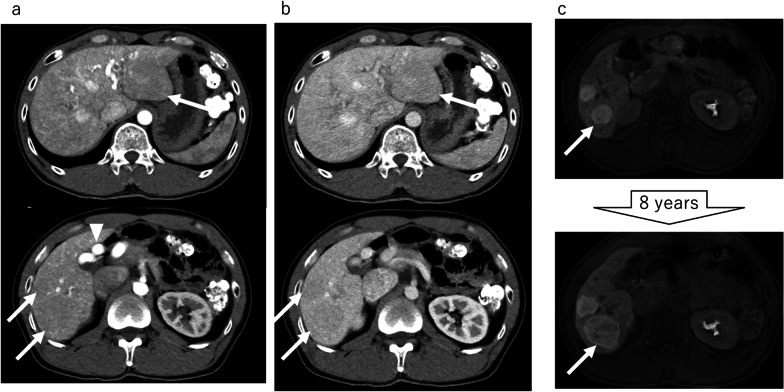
Preoperative images. At the initial visit, CT showed multiple lesions of up to 75 mm in both lobes of the liver (arrows). In the arterial phase, the common and proper hepatic artery (arrowhead) was markedly dilated and tortuous. The lesions were slightly enhanced, and the liver parenchyma was strongly enhanced from the arterial phase and was very heterogeneous due to small telangiectasias (a). In the portal phase, the lesions showed delayed enhancement (b). MRI showed that lesions were enhanced in the hepatocellular phase. During 8 years of follow-up, the only lesion to increase in size was a segment 6 lesion (arrow); this tumor increased in diameter from 30 to 50 mm (c)
